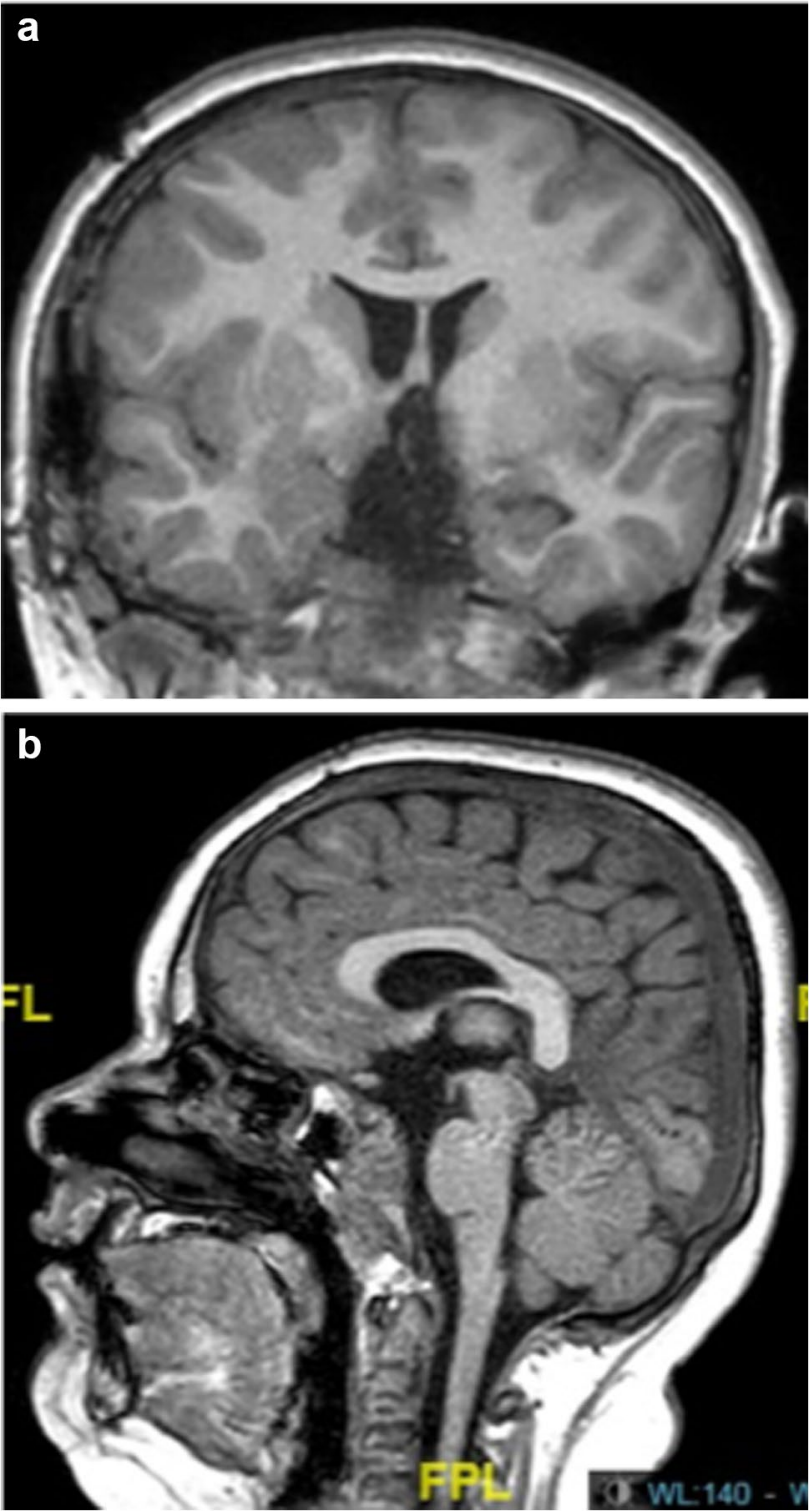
a and b Post-operative MRI showing the complete removal of the tumor
